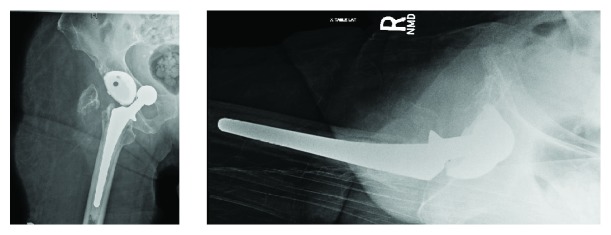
Anteroposterior (AP) and lateral right hip radiographs, status post attempted closed reduction revealing right iatrogenic obturator hip dislocation with femoral component intrapelvic migration.
